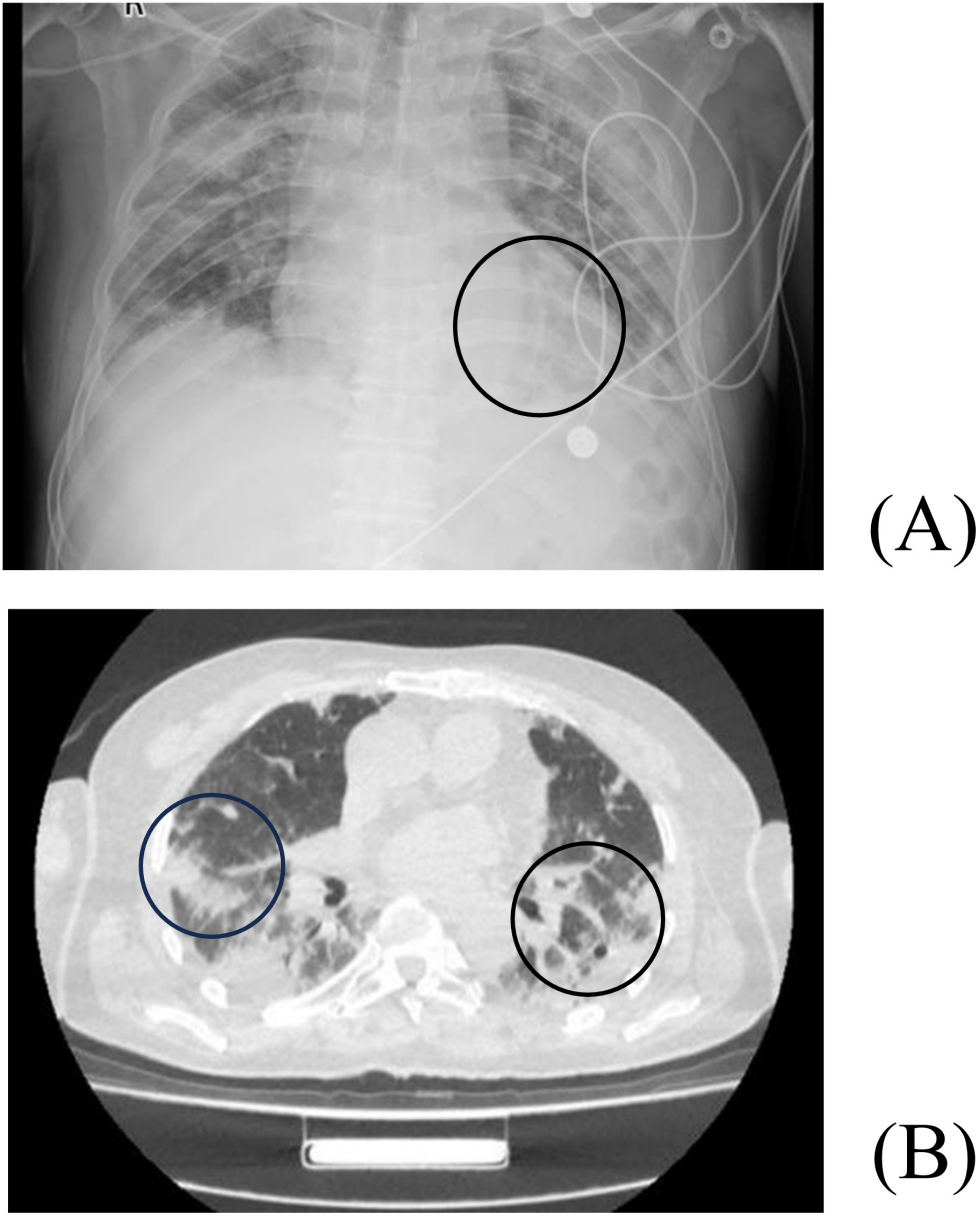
Chest X-ray and CT Findings in Case 2 before treatment. (A) X-ray examination reveals increased and blurred pulmonary markings bilaterally. Patchy areas of increased density with indistinct margins are visible in both lungs. The cardiac silhouette is normal in size, and the costophrenic angles are obscured bilaterally. (B) CT scan reveals multiple inflammatory lesions in both lungs.
